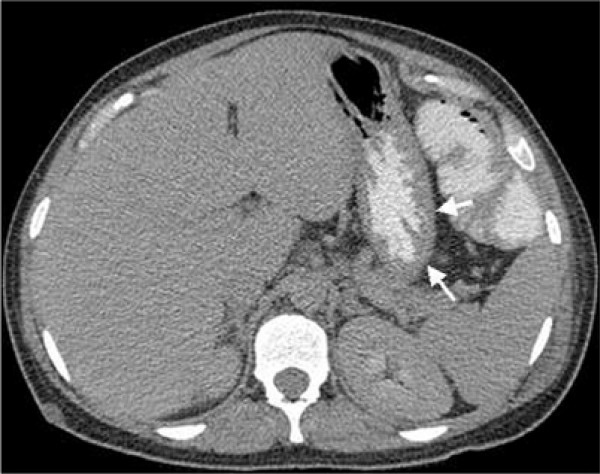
Follow-up abdominal CT on day eight showing resolution of stomach wall and portal venous air.
